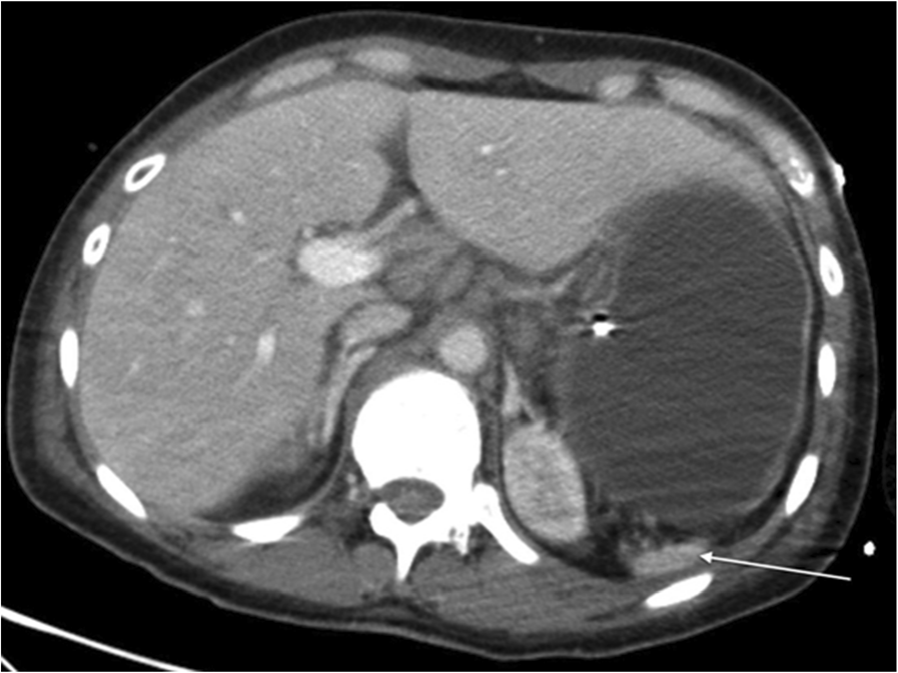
Computed tomography of the abdomen showing splenic atrophy (arrow).
